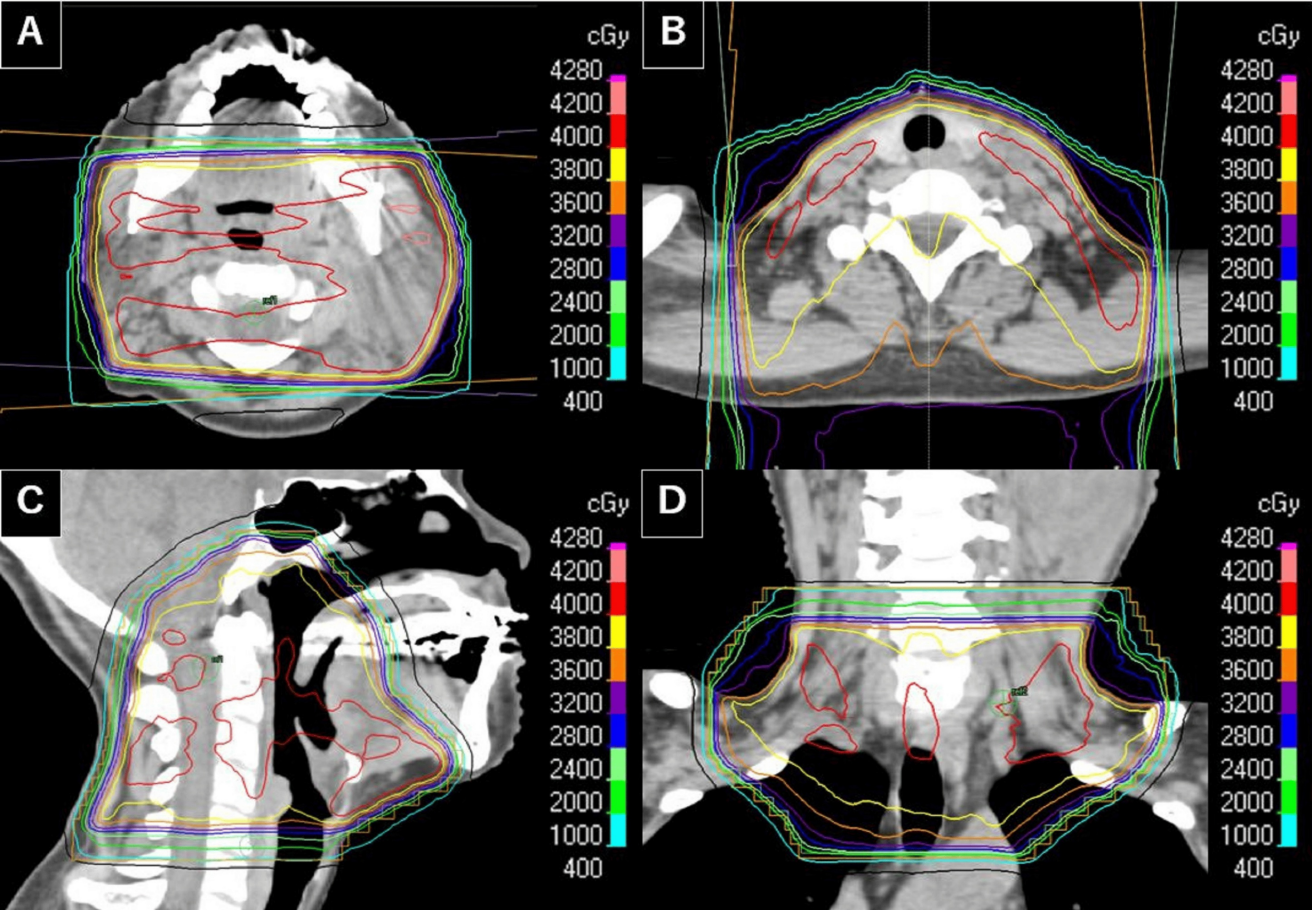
The dose distribution of whole-neck irradiation using the half-beam technique (A-D), delivered with 6 MV X-rays. Axial (A, B), sagittal (C), and coronal (D) CT images show the planned radiotherapy fields and dose gradients for comprehensive neck coverage. Isodose lines are indicated according to the dose scale (right panel). The 40 Gy line is depicted in red, the 28 Gy line in blue, and the 10 Gy line in light blue. The superior border of the irradiation field extended to the skull base including the nasopharynx; the anterior border was set anterior to the retromolar trigone and submandibular region; the posterior border covered the sternocleidomastoid muscle posteriorly including the spinal accessory nerve region; and the inferior border extended to the supraclavicular fossa.
